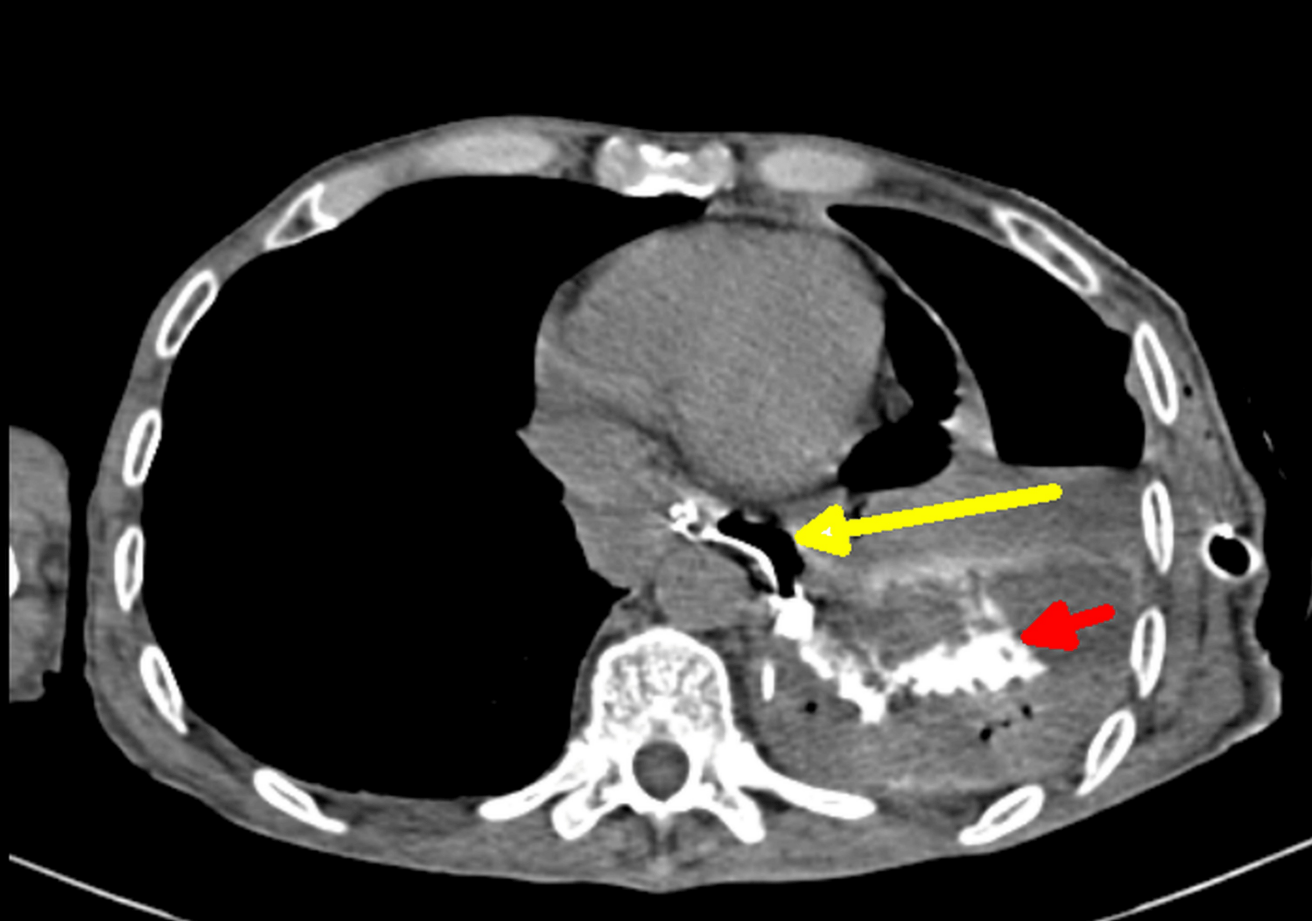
CT with oral contrast scan of thorax with oral contrast showing esophagopleural fistula tract (yellow arrow) and collection of oral contrast in the pleural cavity (red arrow) CT, computed tomography
